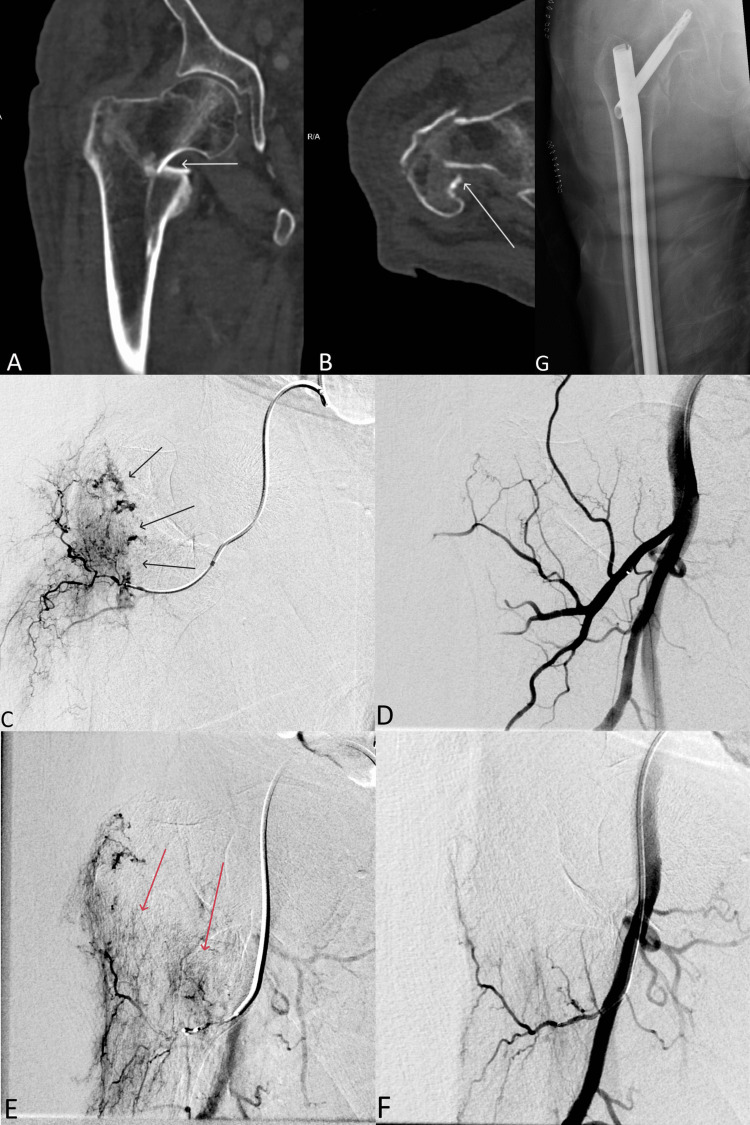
A 78-year-old male with right hip pain. A) B) Coronal and Axial CT of the hip demonstrating an intertrochanteric fracture (white arrows). C) E) Digital subtraction angiography (DSA) demonstrating the areas of tumor blush supplied by the right lateral circumflex (black arrows) and perforating femoral (red arrows) arteries. D) F) DSA demonstrating embolization results. G) Pain film following orthopedic surgery.
